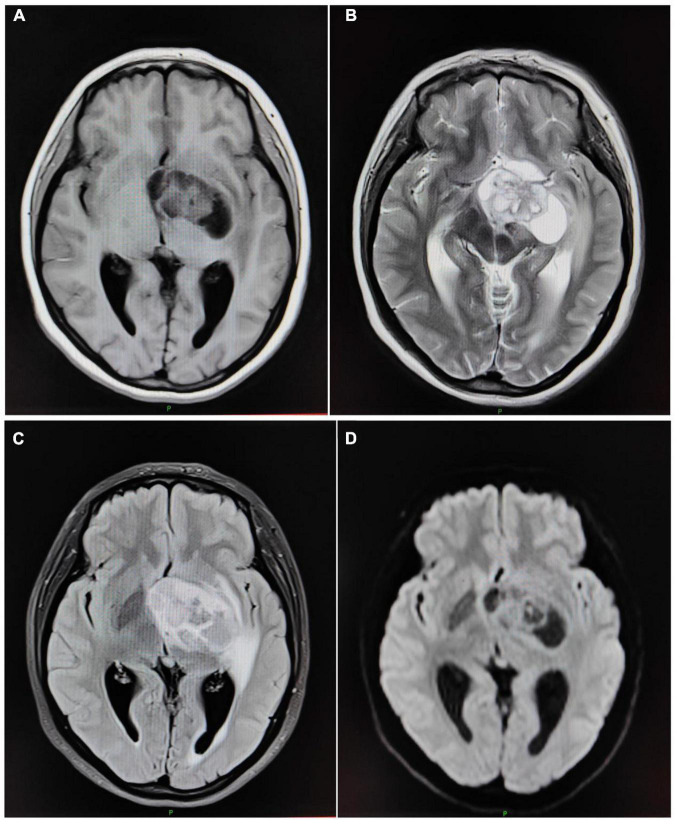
MRI findings of GL. The patient, a 62-year-old woman, presents with multiple, quasi-circular gliomas on the left side of the third lateral ventricle. (A) Transverse T1WI. (B) Transverse T2WI. (C) Transverse T2 FLAIR. (D) Transverse DWI.
